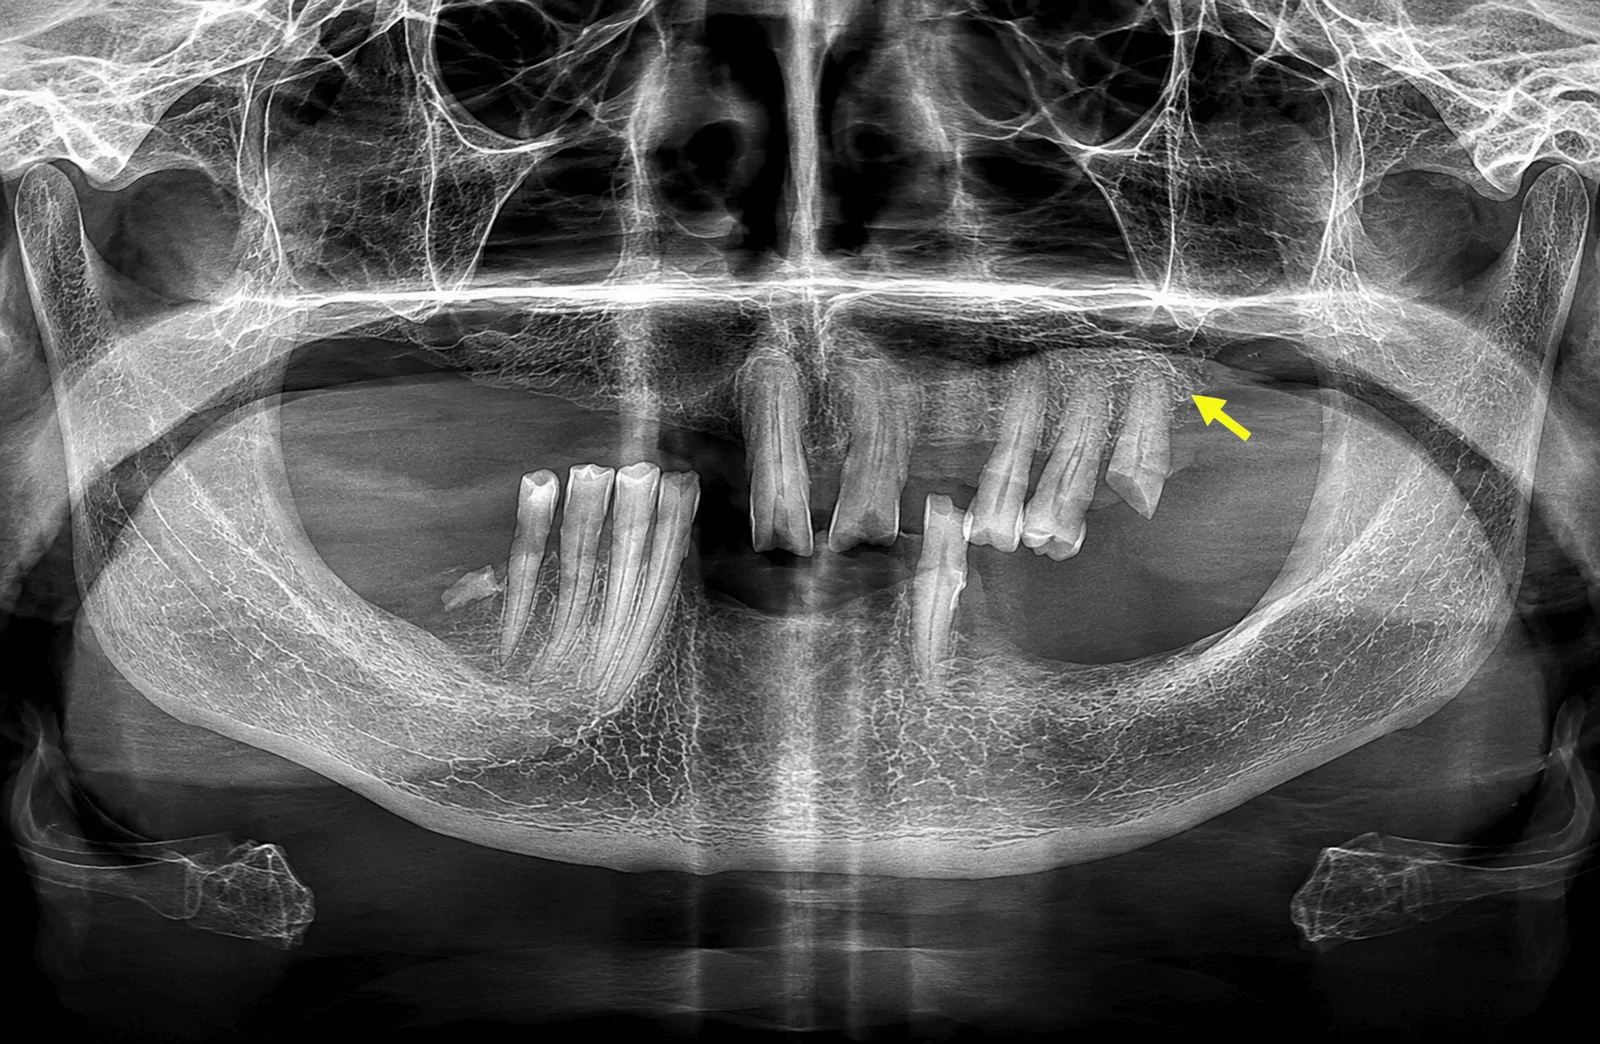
Case two - orthopantomography revealed a carious root stump in the left maxillary second premolar (yellow arrow indicates the infected carious tooth)
